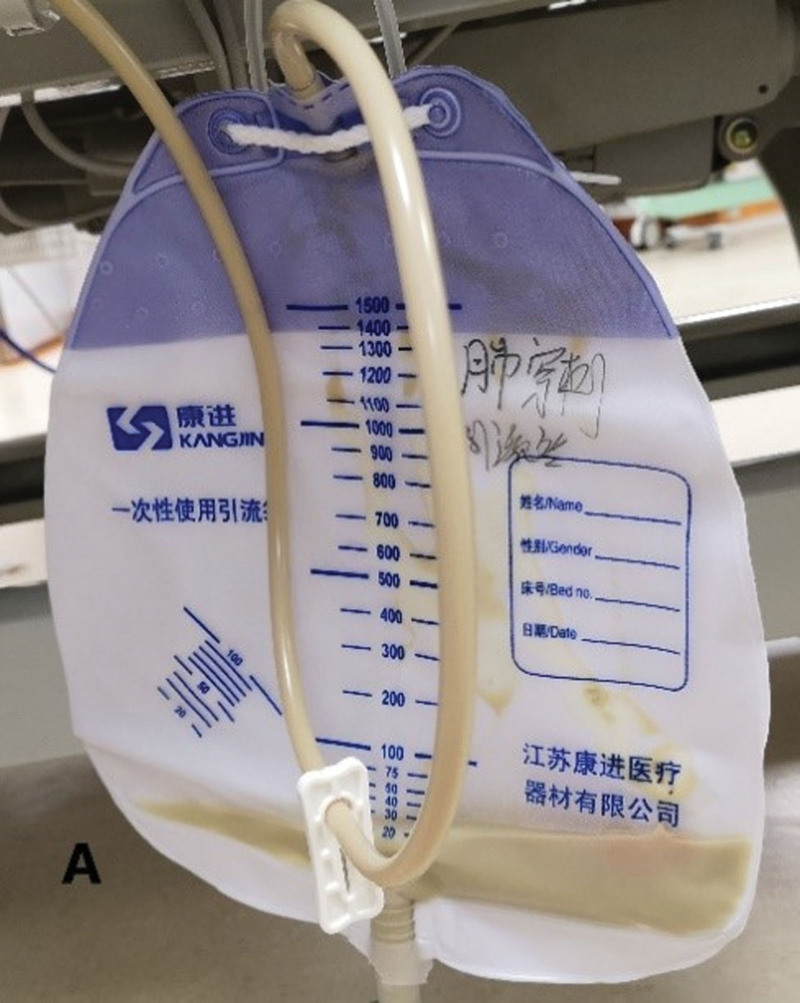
CT-guided puncture for drainage of the lung abscess.
